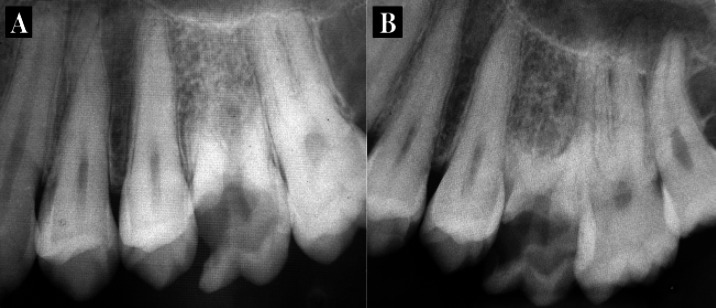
A) Periapical image of left maxillary first molar showing extensive coronal caries and severe root resorption in all three roots; B) A distal angulation showing extensive coronal caries and severe root resorption in all three roots
